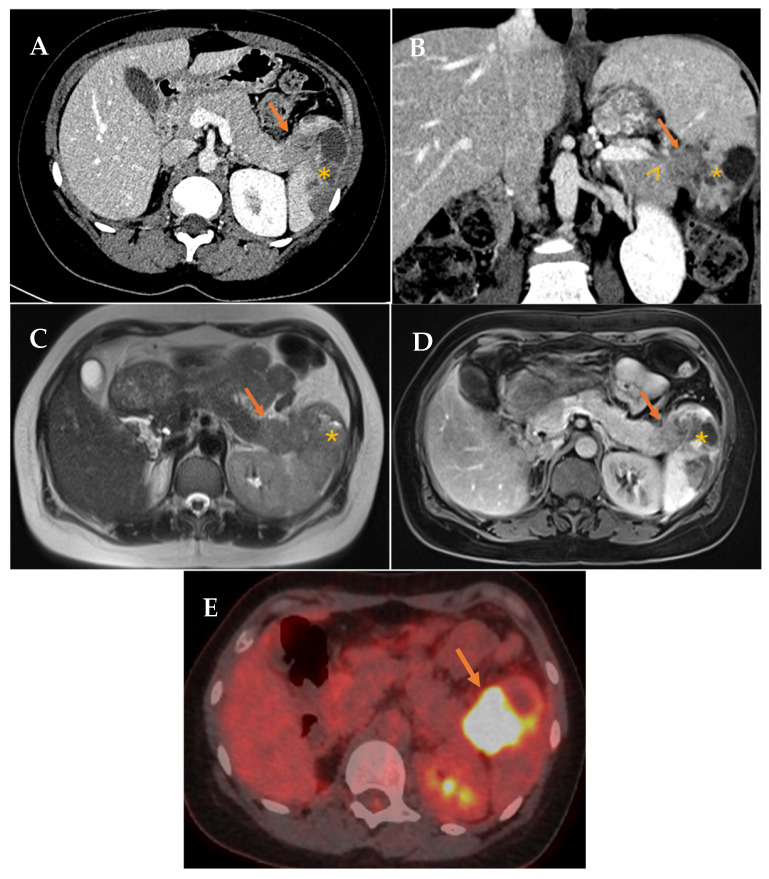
(A,B) Contrast-enhanced CT in portal phase. Axial and coronal reconstruction. Defined hypodense solid mass in the tail of the pancreas (arrow) with infiltration of the splenic hilum (arrowhead). Associated low attenuation changes in the spleen, secondary to infarction (asterisk). (C,D) Axial MRI. T2 and contrast-enhanced T1 sequences. Solid mass in the tail of the pancreas (arrow). Associated signal changes in the spleen, secondary to infarction (asterisk). (E) 18F-FDG PET/CT (18-fluorodeoxyglucose). Mass in the tail of the pancreas-splenic hilum region with pathological FDG uptake with splenic infiltration (arrow).
